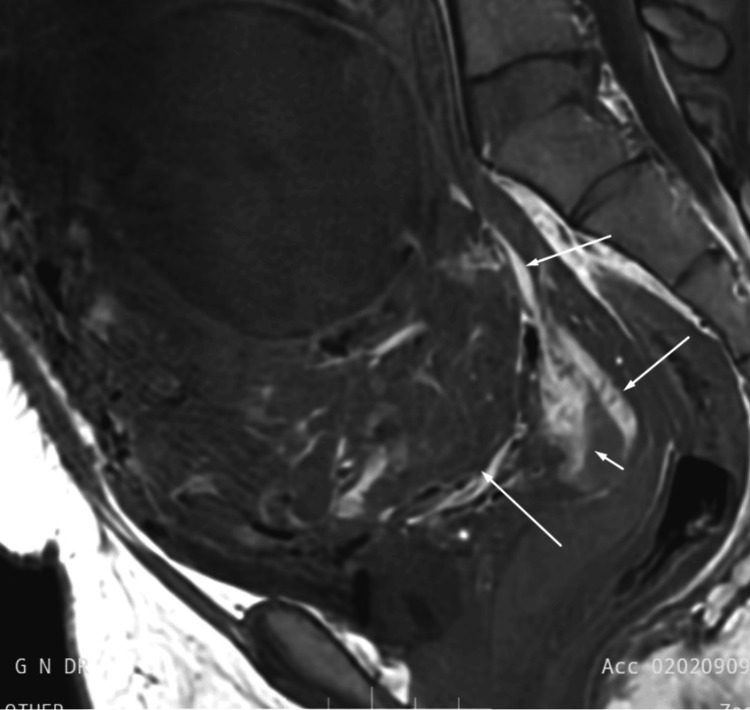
Sagittal T2-weighted MRI image showing loss of retroplacental clear space with severe thinning of myometrium (arrows) in a case of placenta previa This image corresponds to case number 6 in Table 1.
